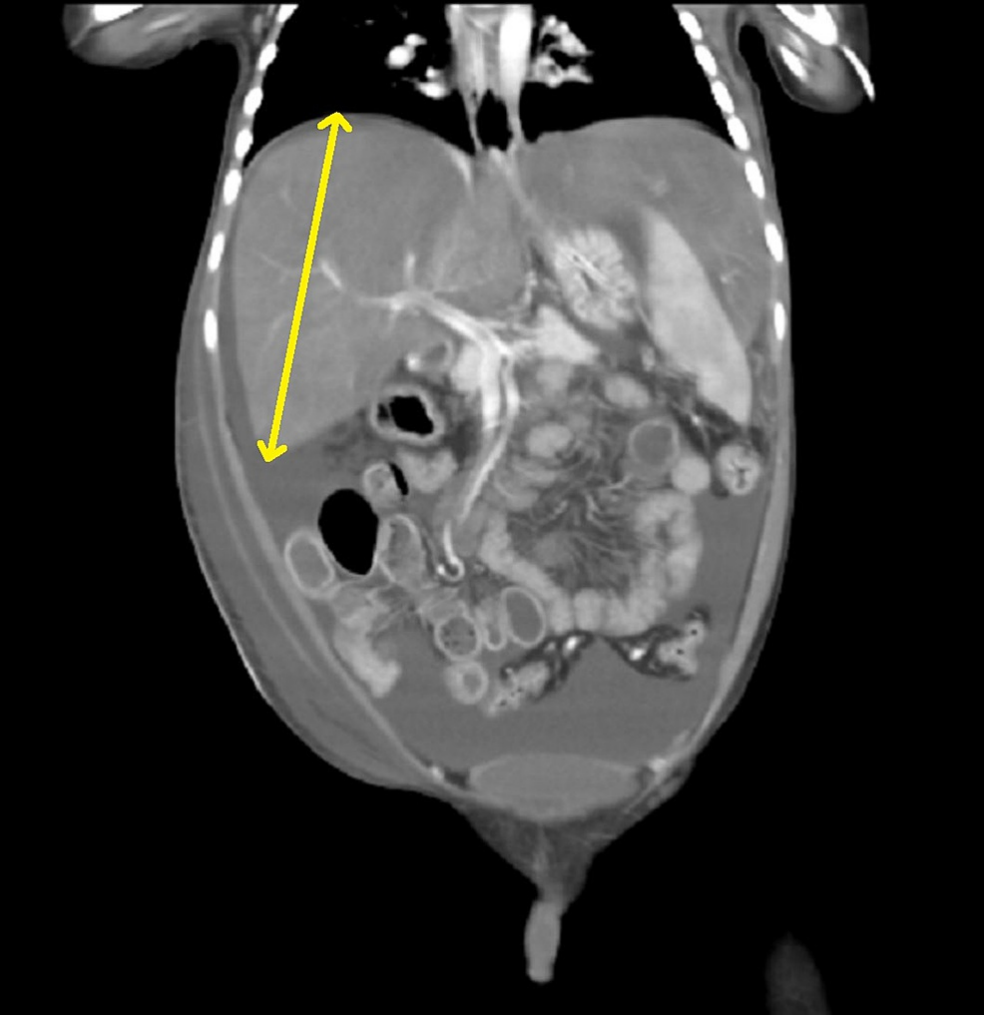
Coronal contrast triphasic CT scan showing massive hepatomegaly with homogenecity (yellow arrow). CT, computed tomography
